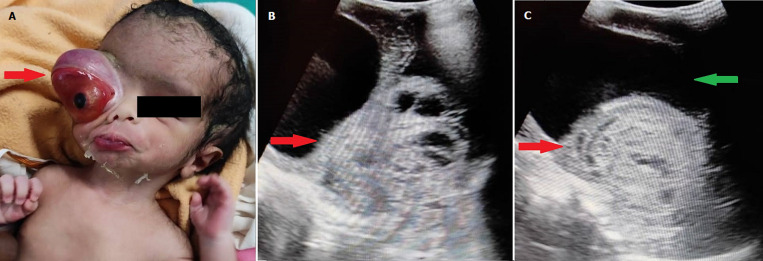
A) severe right eye proptosis with eyeball pushed out (red arrow); heterogeneous lesion with multiple anechoic cystic areas (red arrow); B) ultrasound images; C) heterogeneous well-defined lesion (red arrow), globe is pushed anteriorly (green arrow)
